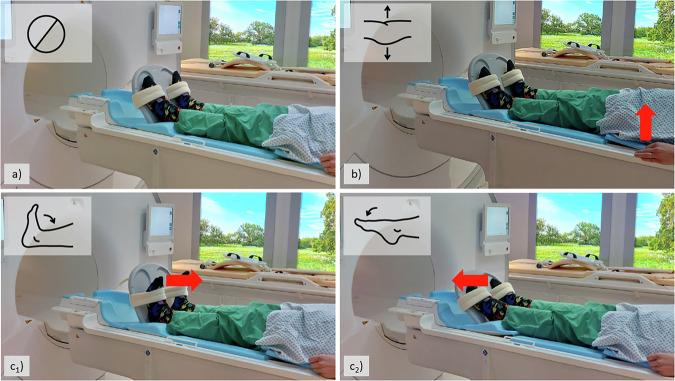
Standardized motion patterns for generating motion artifact-degraded MR images for the test set. Five axial T2-weighted non-fat-saturated 2D turbo-spin echo sequences were acquired consecutively under different conditions: with participants lying as still as possible (a), performing breath-synchronized repetitive (unilateral, yet alternating) gluteal contractions and relaxations (b) and breath-synchronized repetitive dorsiflexion (c_1_) and plantarflexion (c_2_). Red arrows indicate the direction of motion
